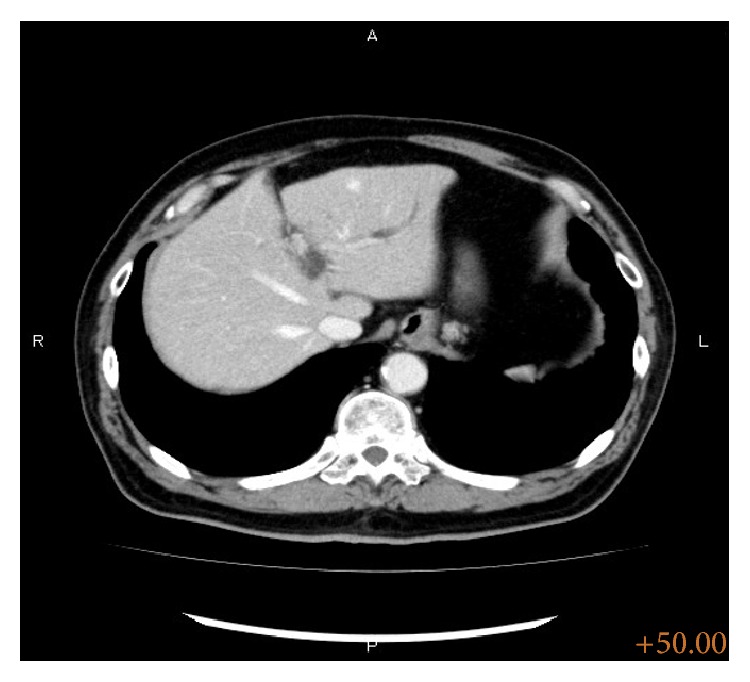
Enhanced CT examination revealed dilatation of the intrahepatic bile duct in the left hemiliver.
